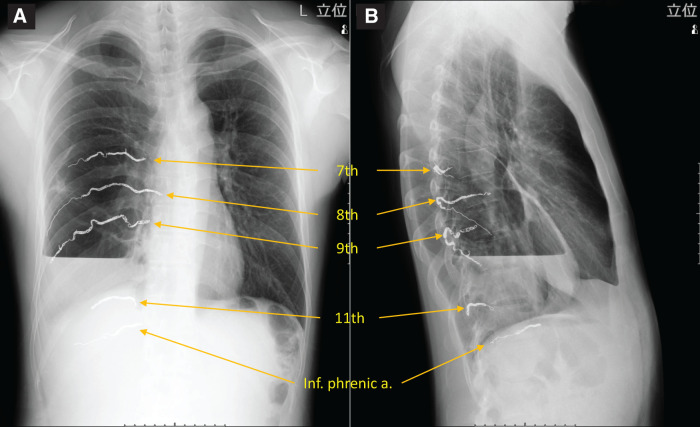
(A, B) Chest radiography after arterial embolization of the 6–11th intercostal and Inf. phrenic as. Embolic microcoils are visible, but gelatin sponge pledgets cannot be detected. Inf. phrenic a., inferior phrenic artery
